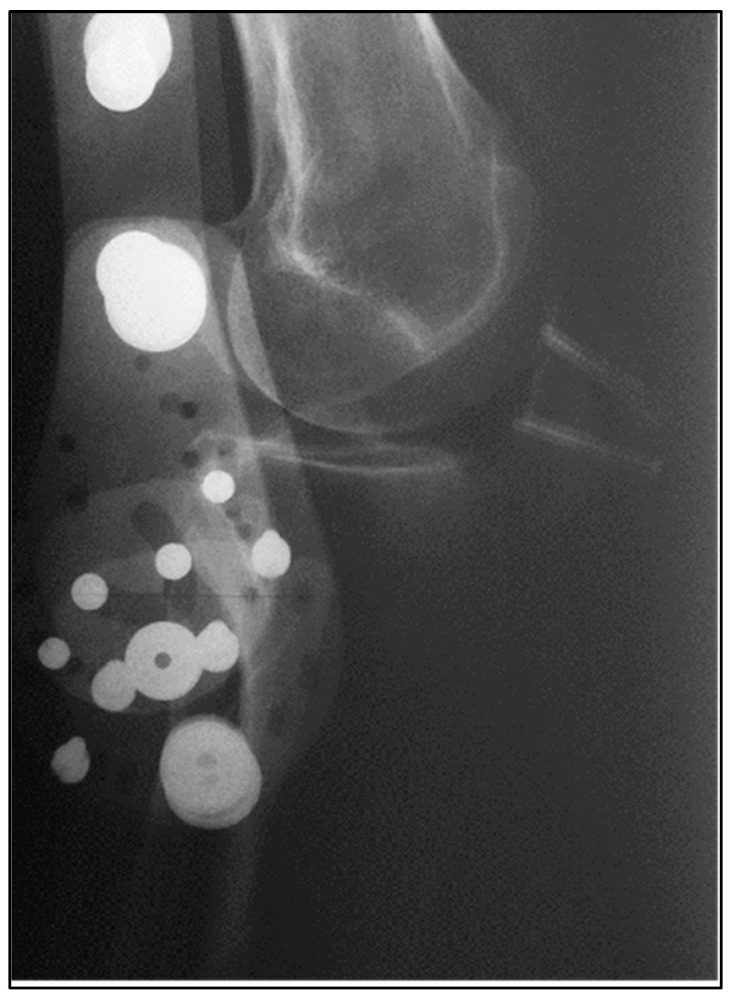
Postoperative radiograph—lateral knee incidence.
